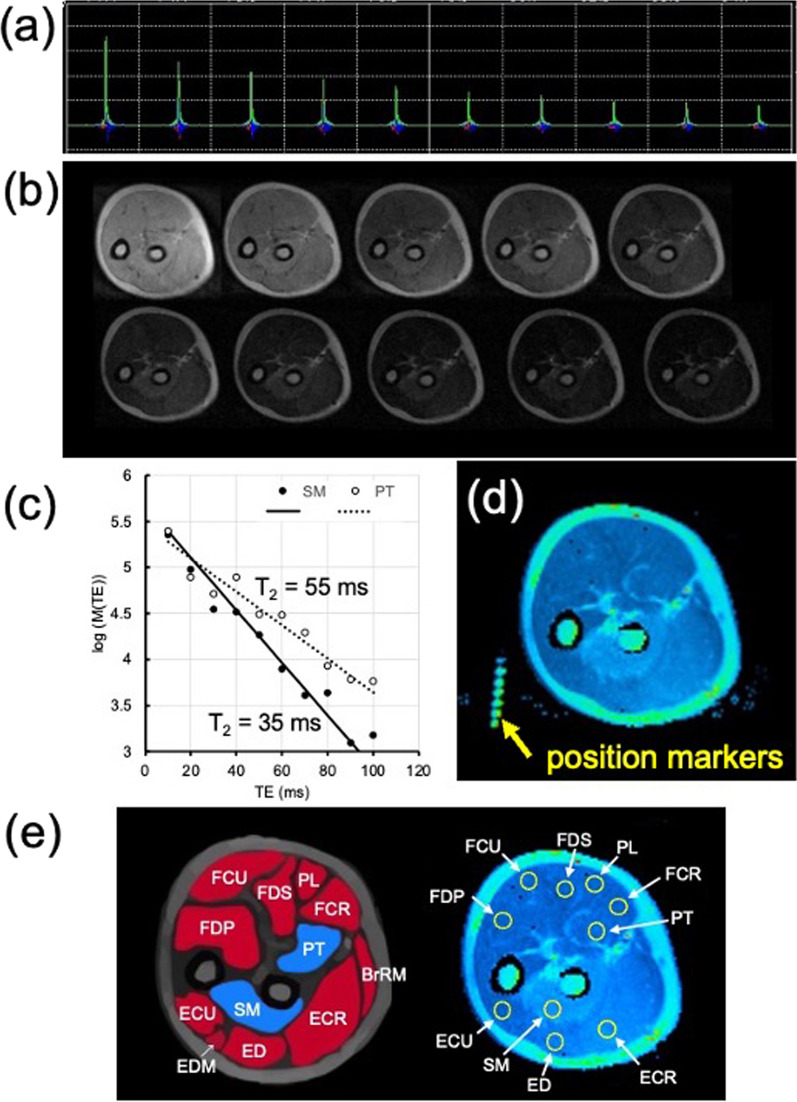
T_2_-map MRI. a Ten spin-echo signals at echo-time (TE) from 10 to 100 ms with a 10 ms step of the transverse MRI of the forearm. b T_2_-weighted MRI of the transverse slice of the forearm at TE from 10 to 100 ms with a 10 ms step shown from the upper-left to the lower-right. c Results of the nonlinear fitting image intensity of the pronator teres (PT: open circle) and supinator (SM: closed circle) after the supination exercise to a function of M(TE) = Mo exp(− TE/T_2_), where M(TE) and Mo are image intensities at a given TE and at TE = 0, respectively. In order to present the single exponential decay, the T_2_ relaxation decay was presented using a semilogarithmic scale. d Typical T_2_ maps of muscles after supination exercise. MR position markers are also presented. e Muscle traces in the transverse MRI of the forearm, and the T_2_-map MRI with ROI positions for 10 muscles. The abbreviations employed are shown in Table 1a
